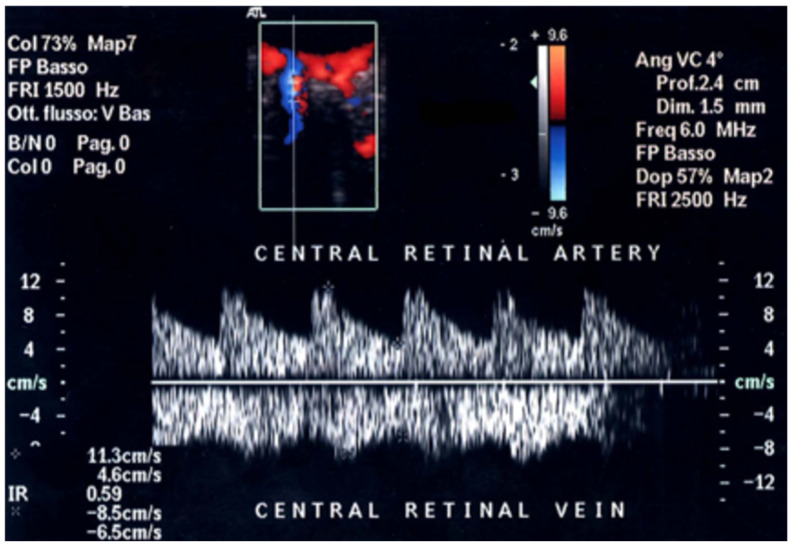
Central retinal artery (CRA) and central retinal vein (CRV), depicted in red and blue respectively, course together within the optic nerve, have a common adventitia and their Doppler signal can be easily distinguished in positive (CRA) and negative (CRV). CRA PSV in normal subjects usually varies from 10 to 16 cm/s, while CRV maxV varies from 5 to 8 cm/s.
